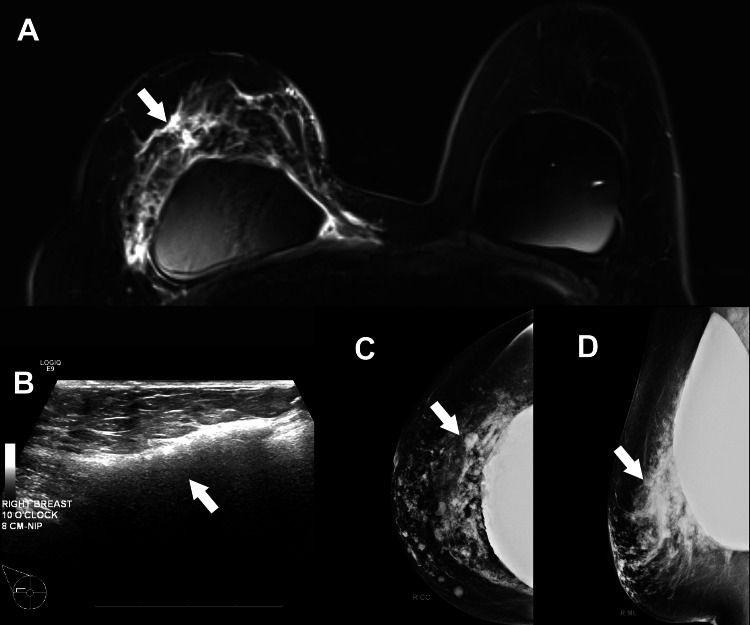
(A) Right breast magnetic resonance imaging showing extensive extracapsular non-mass enhancement. (B) Ultrasound showed multiple areas of snowstorm-type dispersion consistent with free silicone. (C) Craniocaudal and (D) mediolateral oblique mammogram images showing numerous areas consistent with silicone granulomas.
